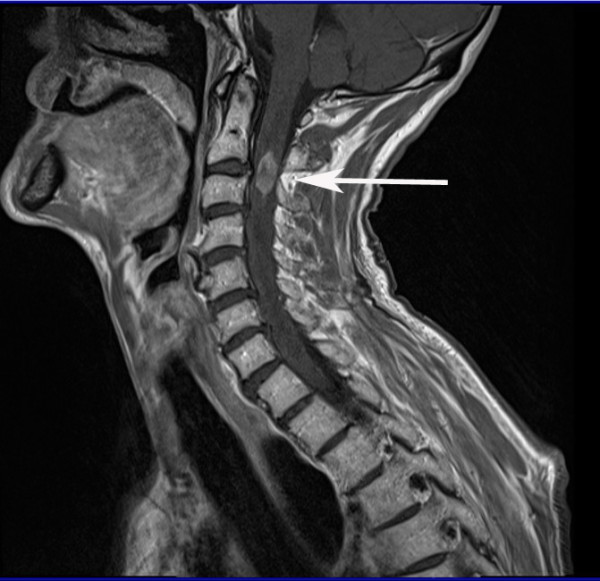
Sagittal T1-weighted MRI of his cervical spine.
